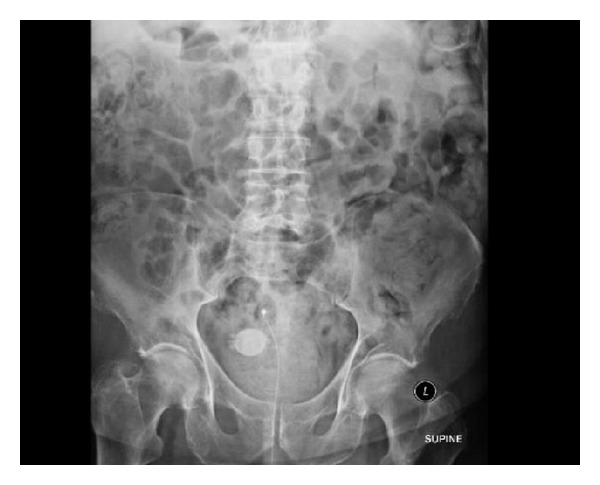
Plain abdominal radiograph clearly showing spiculated bladder calculi.
